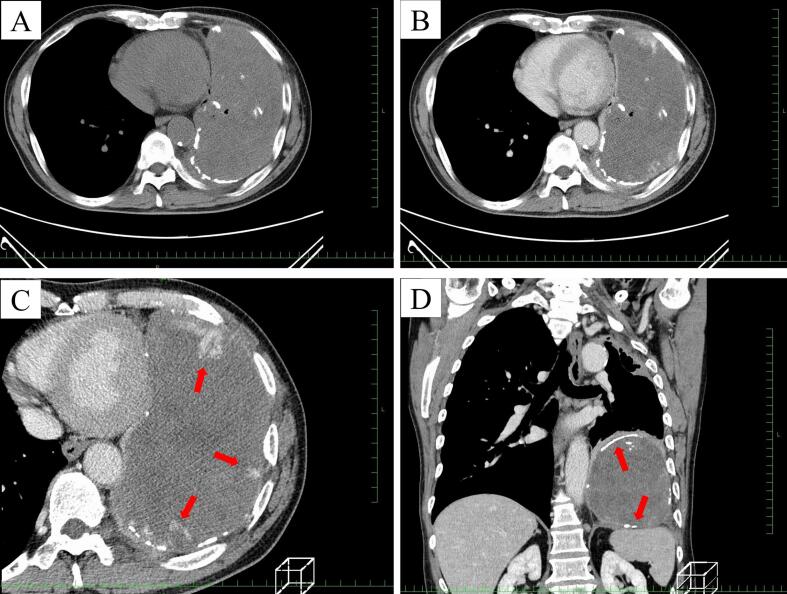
(A) Computed tomographic (CT) scan of the chest showing a large mass with central low attenuation measuring 16 × 12 × 8 cm. (B) Contrast-enhanced CT scan showing non-homogeneous enhancement. (C) Newly formed vessels (red arrows) inside the lesion on the thoracic side. (D) Calcification (red arrows) detected at peripheral lesions of the mass. (For interpretation of the references to colour in this figure legend, the reader is referred to the web version of this article.)
